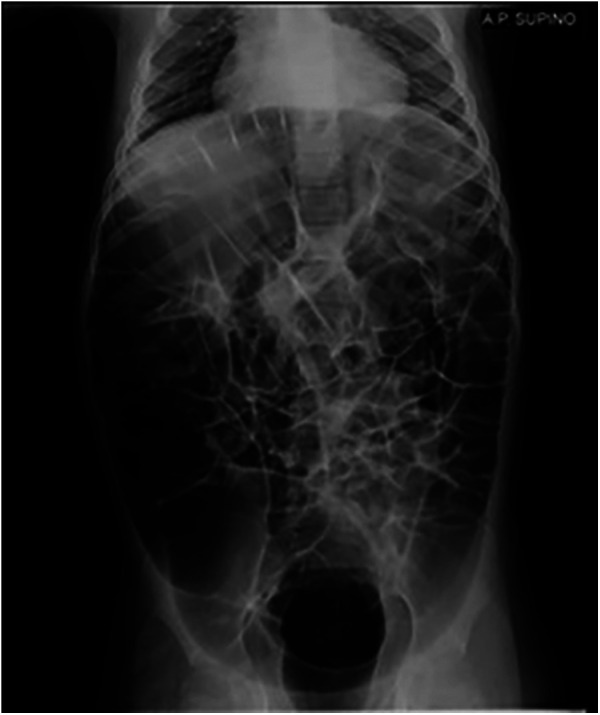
Upright plain abdominal x-ray showing massive dilation of the intestinal loops.
